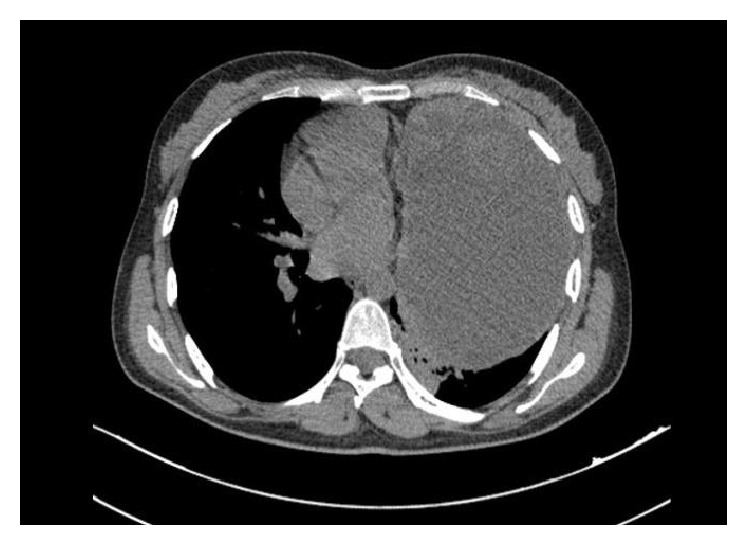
CT scan of chest shows a mass of the left hemithorax, which probably originated in the mediastinum and extended to the whole left pleural space.
